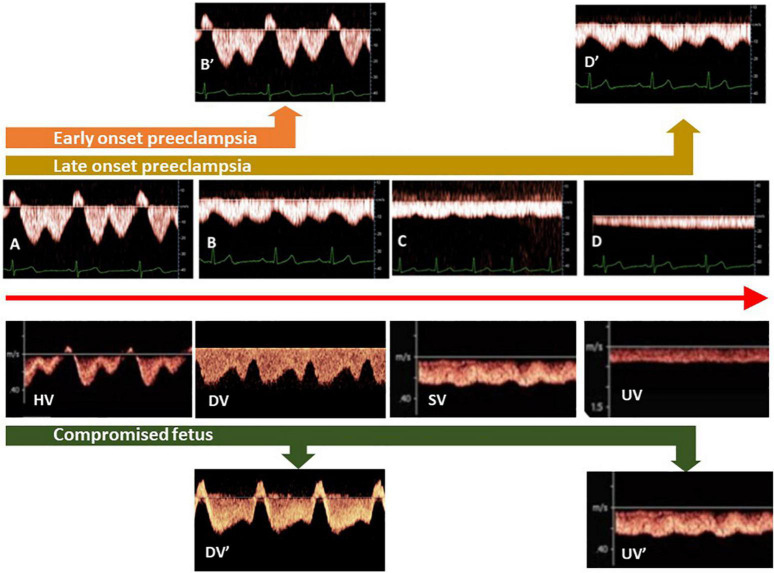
Different types of venous Doppler waveforms in maternal and fetal circulations, varying between triphasic (A), biphasic (B), and monophasic (C) to flat (D). In the fetus and non-pregnant adults, triphasic patterns are found close to the heart [hepatic veins (HV)], whereas flat patterns are present at distant locations [limbs, umbilical veins (UV)]. During uncomplicated pregnancy, HV patterns shift from (A–D). In the clinical stage of preeclampsia with FGR, biphasic HV patterns become triphasic (orange arrow), whereas in term preeclampsia, monophasic patterns evolve to biphasic (brown arrow). In ductus venosus (DV) of FGR fetuses, a shift from biphasic to triphasic patterns occurs simultaneously with deteriorating fetal condition (green arrow). (SV: fetal splenic vein).
